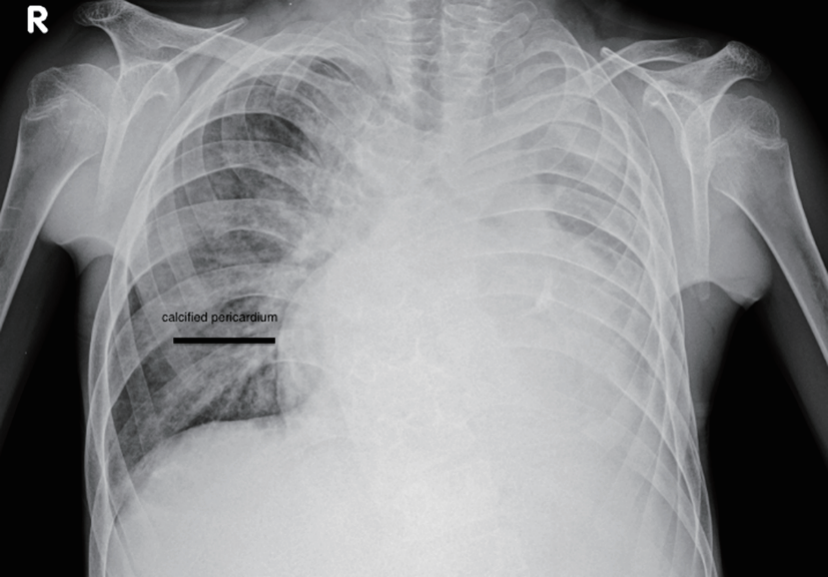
Chest X-ray PA view showing pericardial calcification.
